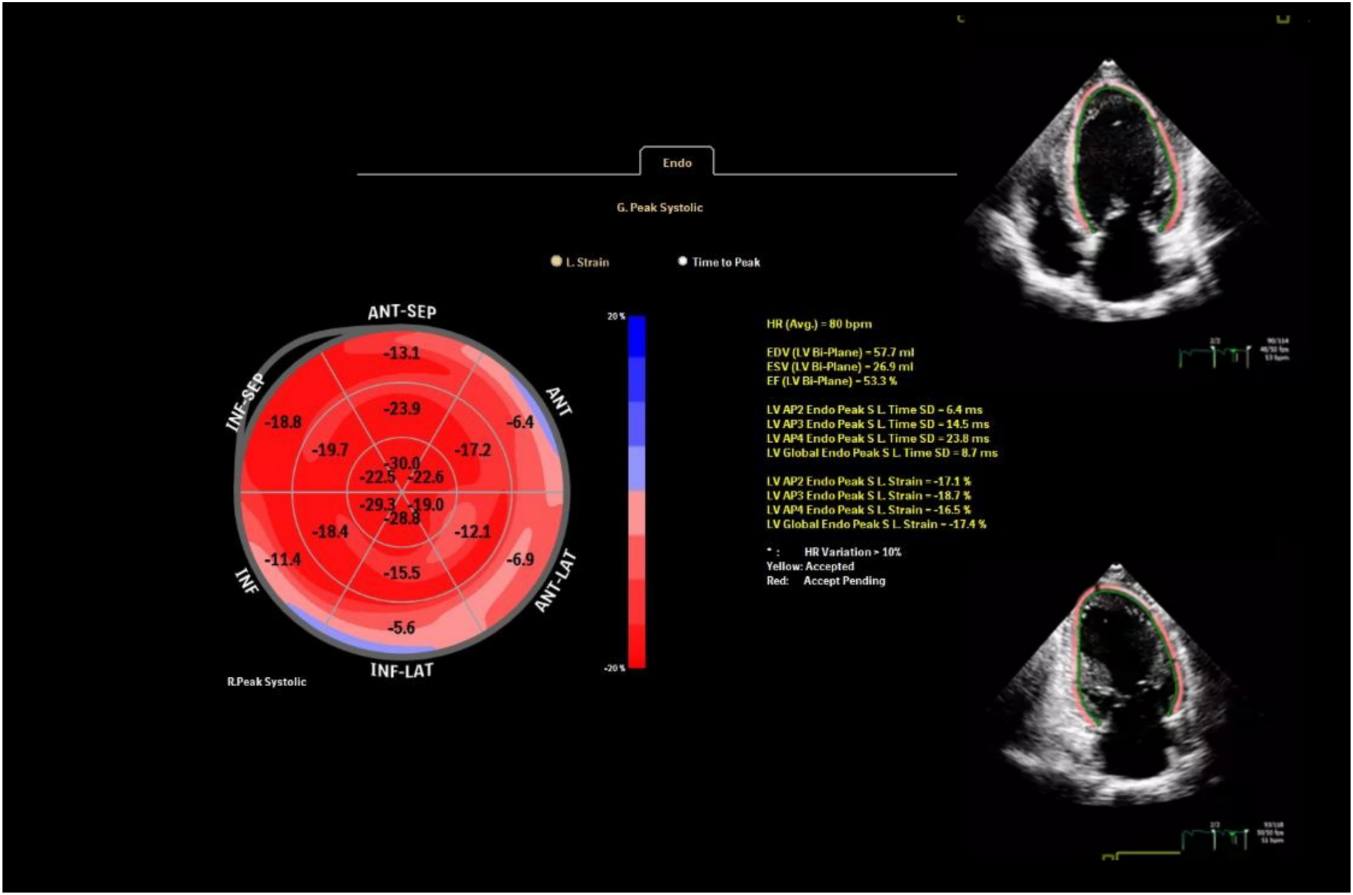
Measurement of global longitudinal strain using speckle-tracking echocardiography.
